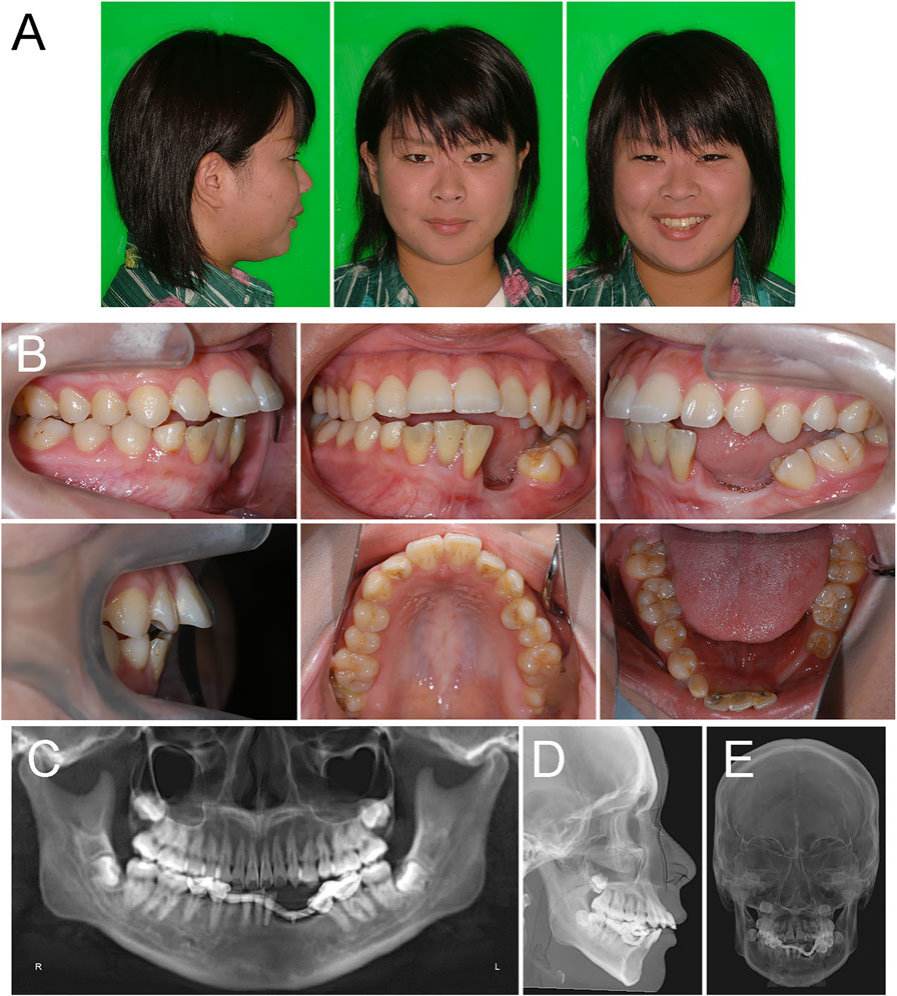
Pre-treatment recordings. a Extraoral photographs. b Intraoral photographs. c A panoramic radiograph. d A lateral cephalogram. e A posteroanterior cephalogram
